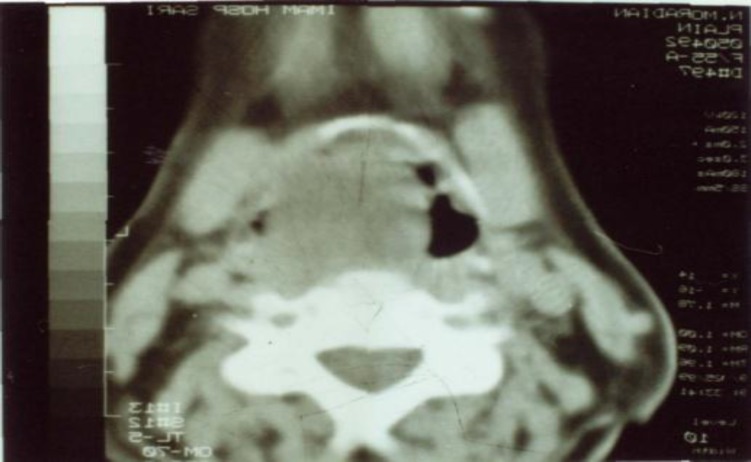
CT scan view of the tumor
